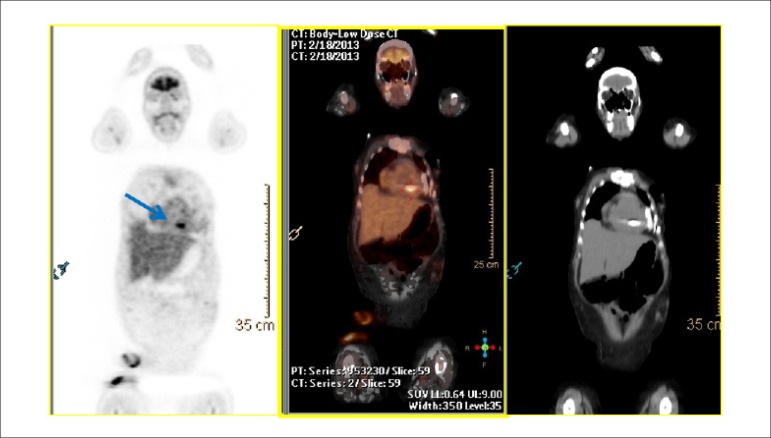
Male patient, age 65, with pacemaker implant 4 months prior (removed due to subcutaneous pocket infection); new implantation with the distal end of the right chambers. He evolved with dyspnea and fever 20 days prior; blood culture was positive for S. aureus. FDG-^18^F - PET/CT study was positive for endocarditis in the implant site; maximum standard uptake value (SUV) = 8.1.
